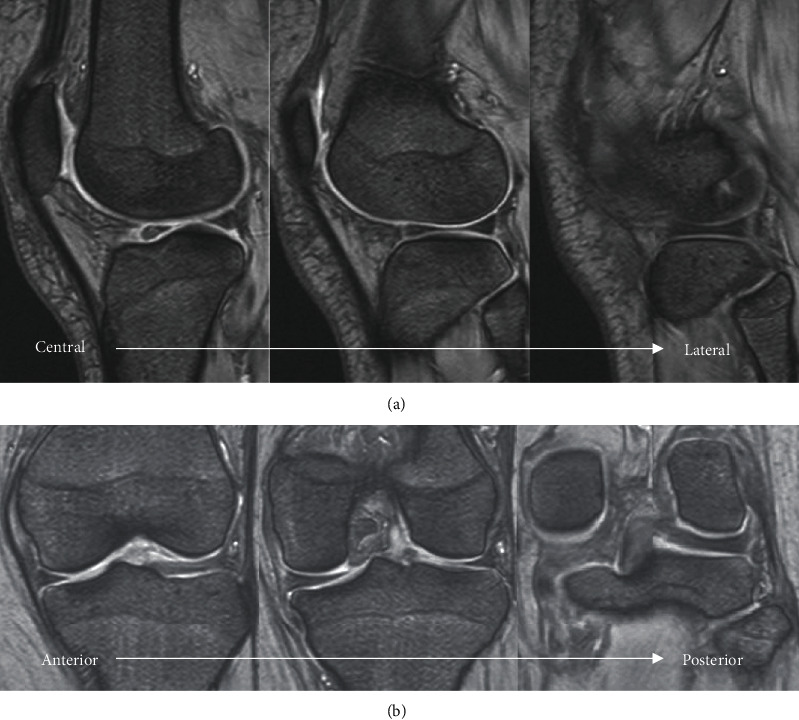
Preoperative MRI. Preoperative MRI showed no abnormal intensity at the meniscus. (a) T2^∗^ sagittal; (b) T2^∗^ coronal. MRI: magnetic resonance imaging.
